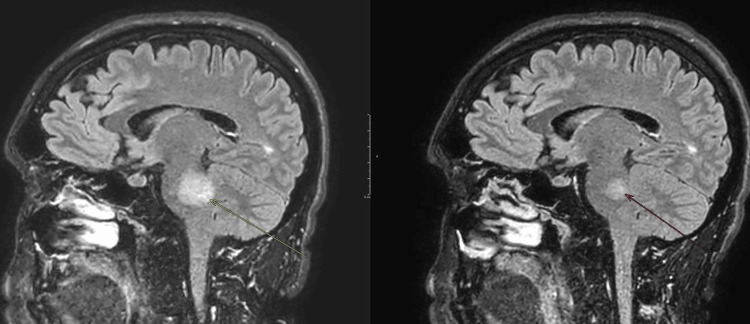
MRI of the brain shows significant expansion of the left pontine lesion, which demonstrates diffusion restriction at its margins. These appearances overall remain in keeping with acute active demyelination.
